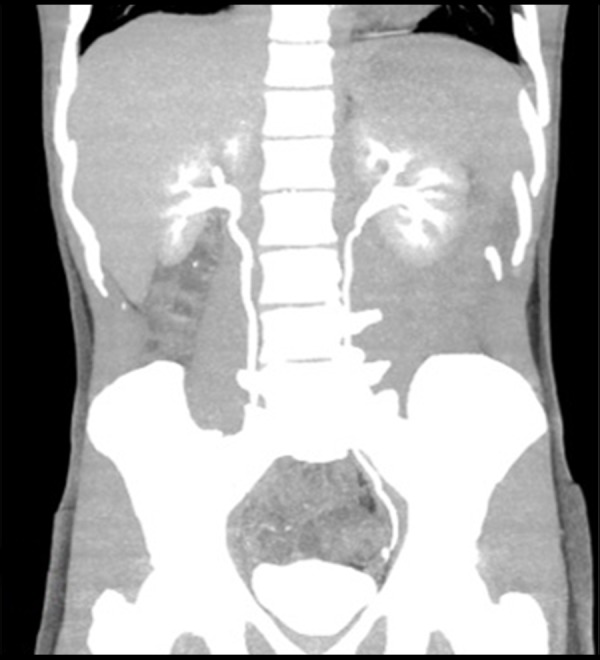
Coronal CT urography image showing no evidence of filling defect in the left upper ureter.
